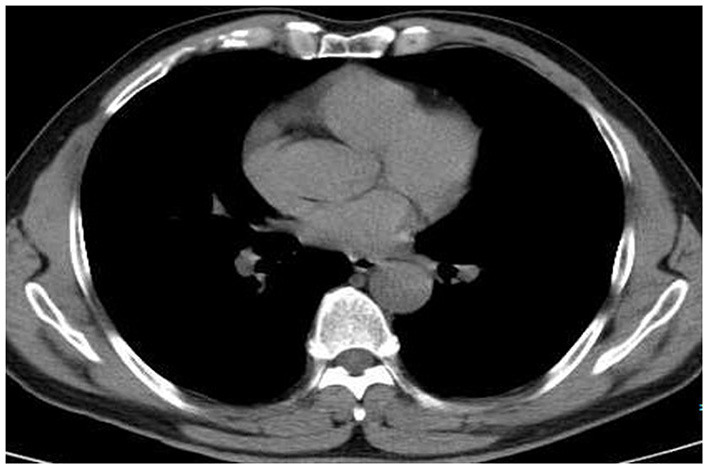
Two months postsurgery, the patient was followed up by chest CT and had no evidence of tumor recurrence.
